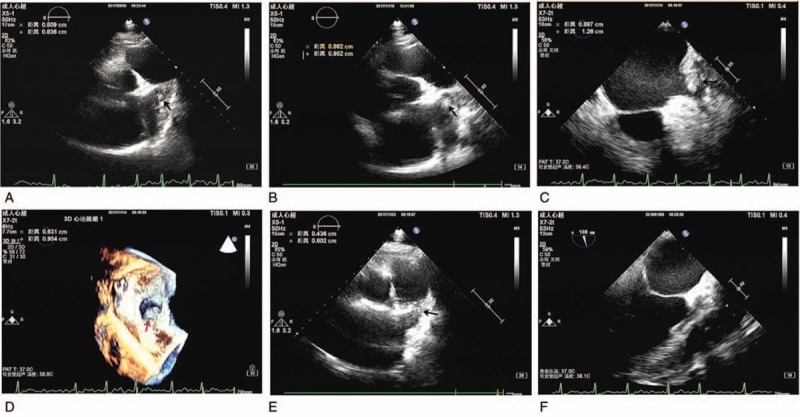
Evidence of a thrombus in the left atrium and its response to non-vitamin K antagonist oral anticoagulant (NOAC) therapy using echocardiography. (A) Transthoracic echocardiography (TTE) showed a thrombus (black arrow) in the left atrium upon initial presentation. (B) TTE imaging after 1-month rivaroxaban treatment revealed the thrombus had increased in size (black arrow). TEE imaging (C) and 3D imaging (D) further confirmed the presence of a left atrial thrombus. (E) TTE imaging showed dabigatran treatment reduced the size of the thrombus, with a later TEE examination revealing complete elimination of the thrombus (F).
